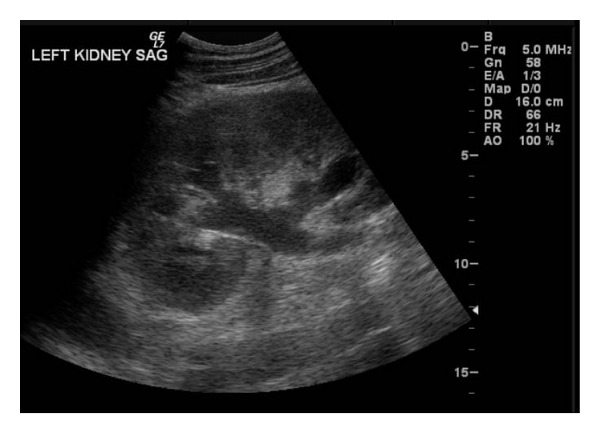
Renal ultrasound demonstrating mild left hydronephrosis.
